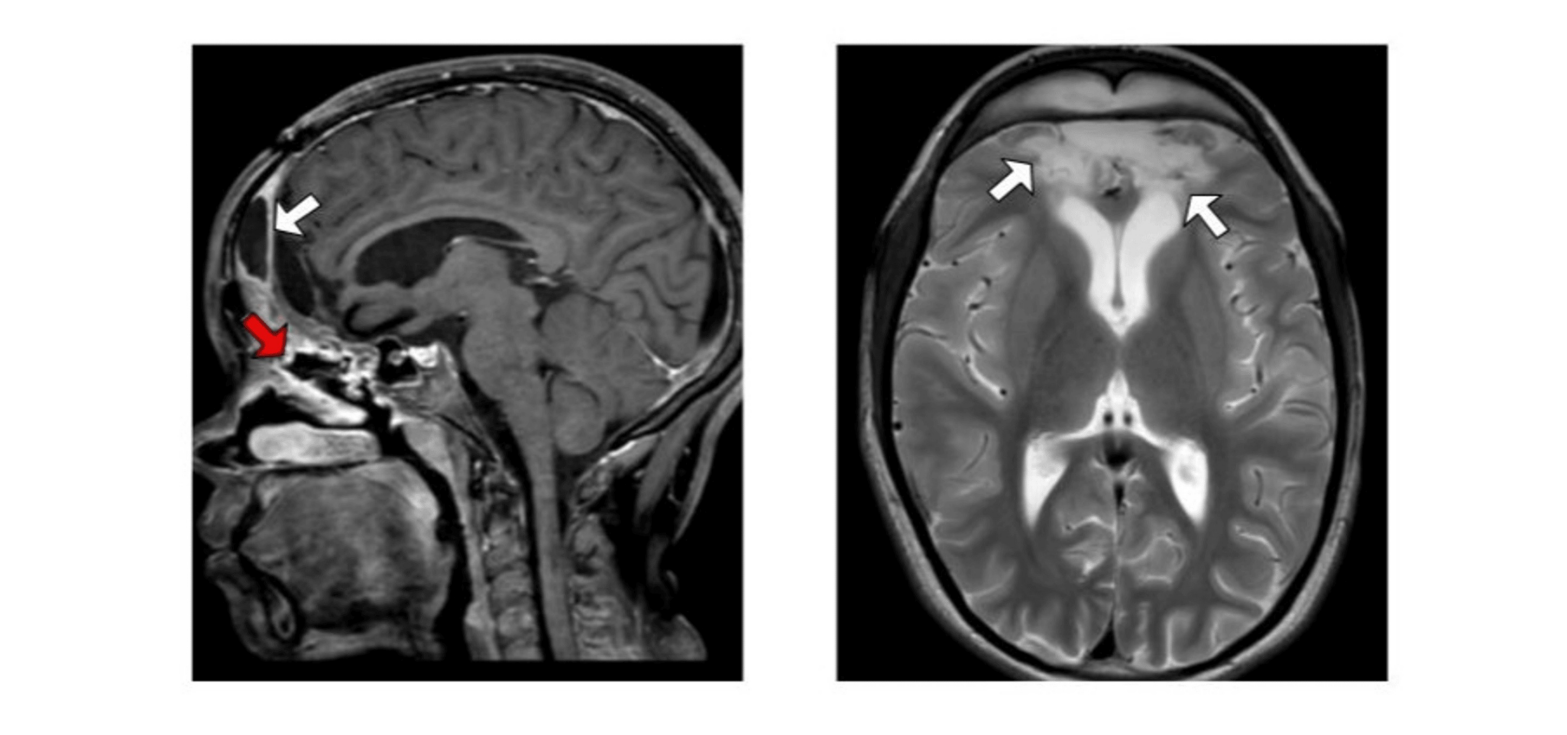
Magnetic resonance imaging of control skulls (four months after hospital discharge) Findings consistent with post-operative changes, following frontal craniotomy; encephalomalacia and cavity in the anterior aspect of the frontal lobes (white arrows); and mucosal thickening of the maxillary and frontal sinuses (red arrow).
